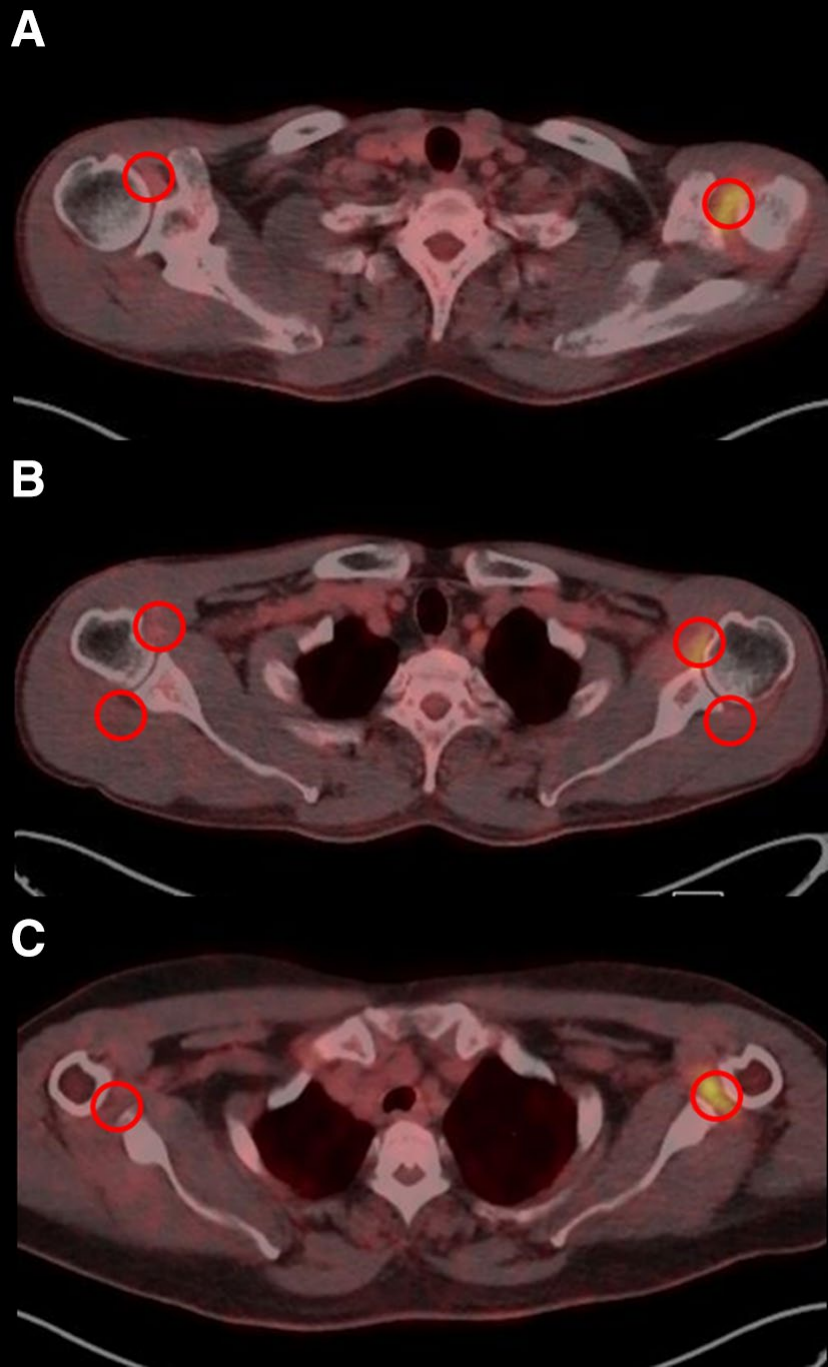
Standardized uptake values measured at the four regions of interest from the attenuation corrected axial images on ^18^F-FDG PET/CT. a Rotator interval. b Anterior and posterior joint capsule. c Axillary recess
